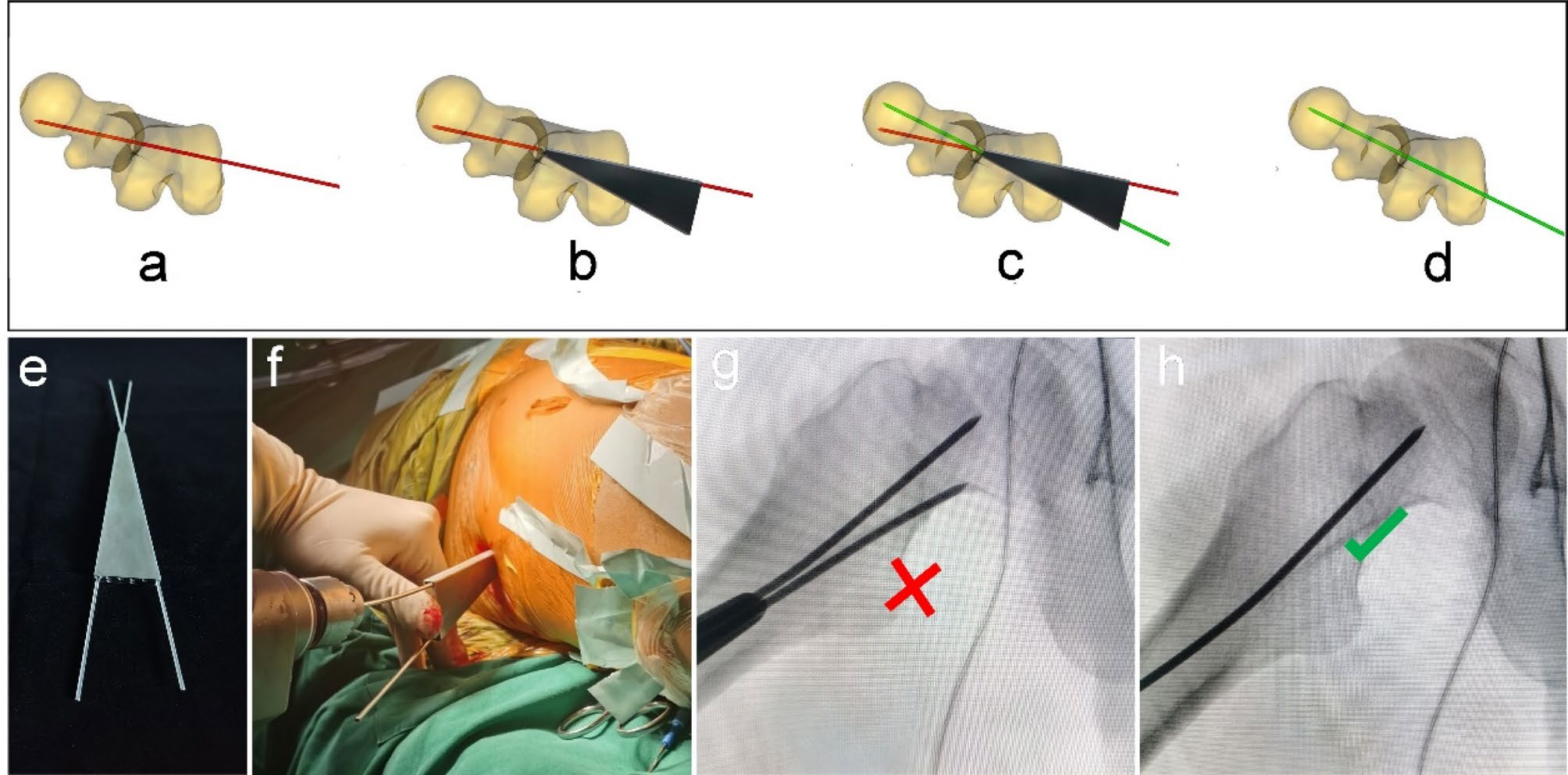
The accurate positioning device accurately located the necrotic parts by the adjustment of femoral anteversion Angle. (a) The Kirschner wire was inserted into the femoral head and neck and positioned inaccurately; (b) The device contacted the bone surface by connecting the Kirschner wire; (c) An additional Kirschner wire was inserted according to the adjustment of angle; (d) The ideal position of the Kirschner wire to be inserted into the necrotic parts of the femoral head; (e) The general appearance of the device; (f) Intraoperative use of the device; (g) Not ideal localization of femoral anteversion Angle of Kirschner wire, using the device and quantitative adjustment of femoral anteversion Angle under X-ray fluoroscopy; (h) Ideal localization of femoral anteversion Angle of Kirschner wire.
